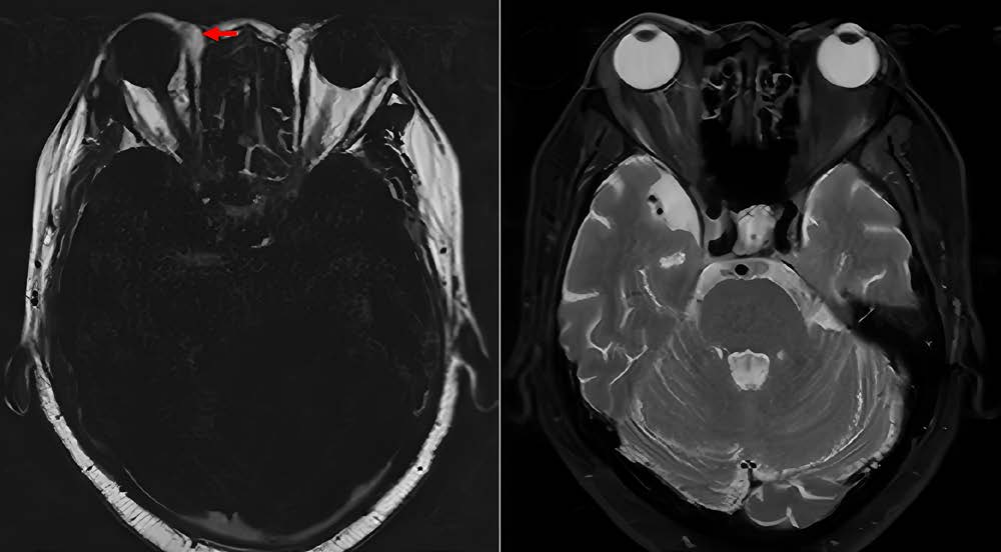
Plain + contrast-enhanced orbital MRI: slight increase in lesions in the space outside the medial and lateral rectus muscles of the right orbit.
